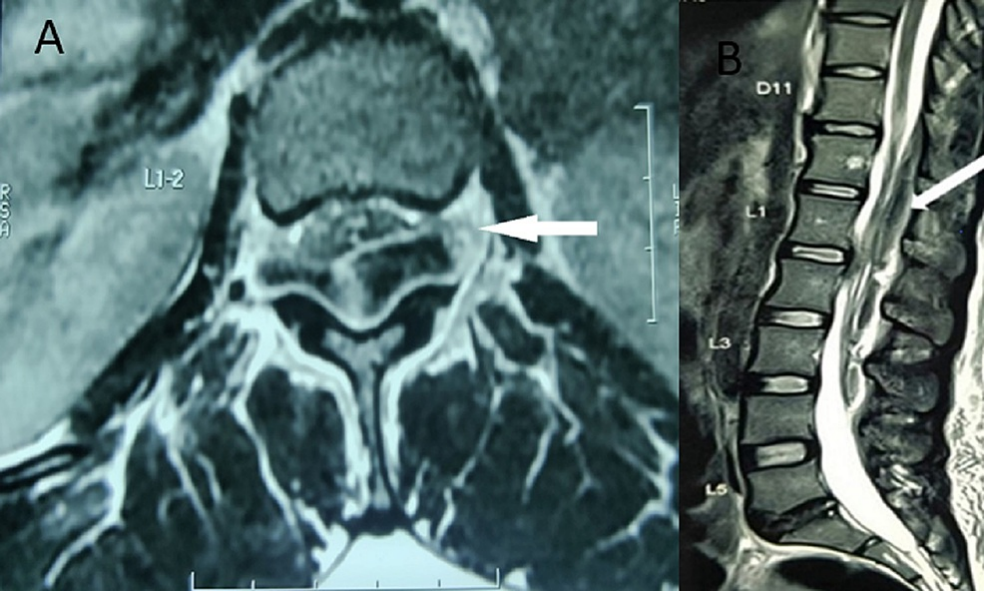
MRI axial (A) and sagittal section (B). T2-weighted images showing hypointense lesion at the thoracolumbar area. The mass is pushing the cord to the anterior side.
